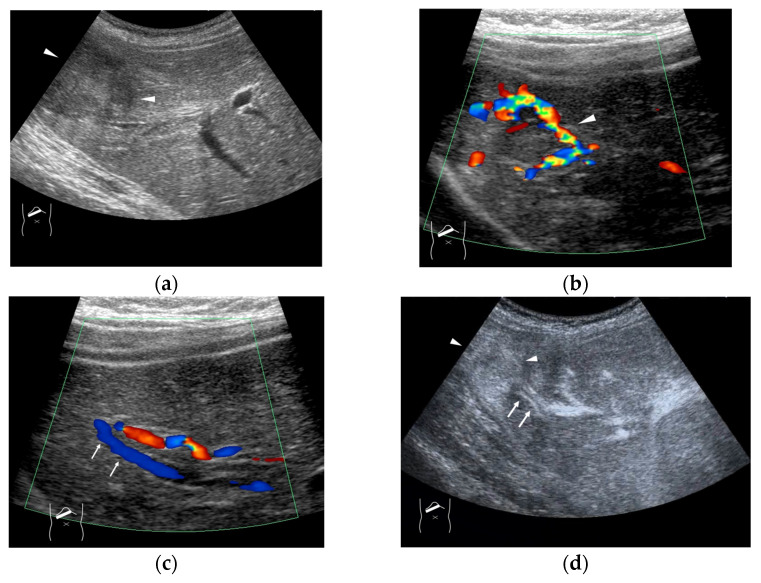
Representative case of surgically resected well-differentiated HCC arising from minimally fibrotic (F1) liver: (a) B-mode reveals HCC in segment 6 (arrow heads). (b,c) Color Doppler shows tumor vascularities in a basket pattern (arrow heads) and a hepatic vein (arrows). (d) CEUS reveals a markedly enhanced HCC lesion (arrow heads). A hepatic vein (arrows) is enhanced immediately after tumor enhancement.
